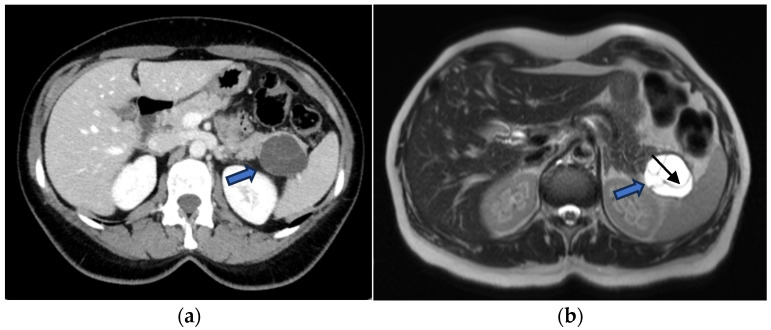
(a) Axial CECT scan of a 50-year-old-woman shows a large (40 mm), multiloculated lesion located in the pancreatic tail (blue arrow); (b) MRI demonstrates a well-circumscribed, multilocular cystic lesion with thick internal septa (black arrow), showing hyperintense signal on T2-w images.
